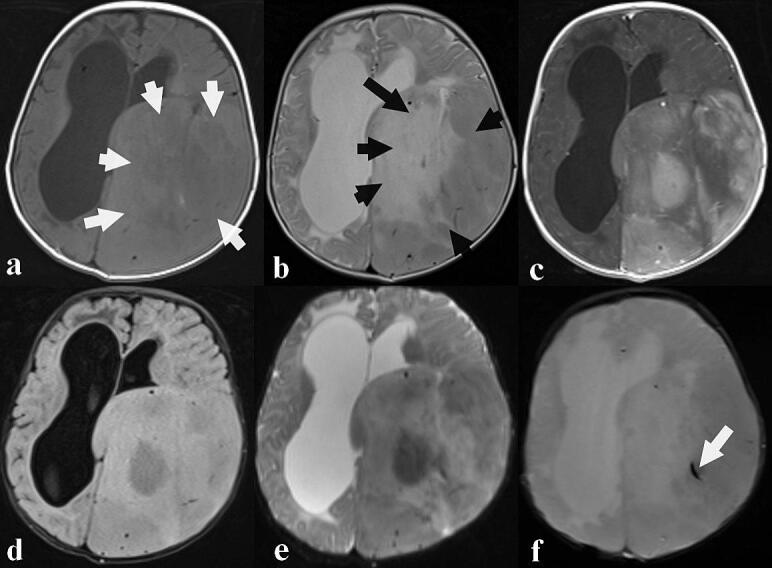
Axial brain MRI showing a large left parieto-occipital space-occupying lesion with mixed signal on T1-weighted sequence (some areas in hyposignal and others in isosignal) (a, white arrows) and of heterogeneous signal on T2-weighted sequence (b, black arrows) and with heterogeneous enhancement after gadolinium chelate injection (c). Note a slight perilesional edema all around on the T2 fluid attenuated inversion recovery sequence (d). The tumor was compressing the midbrain, cerebral aqueduct and the third ventricle and which is responsible for a significant right lateral ventricle dilatation and moderate frontal horn of the left lateral ventricle dilatation. There was no hypersignal on diffusion image (e) and the T2 sequence in gradient echo showed some stigmata of bleeding (f, white arrow).
